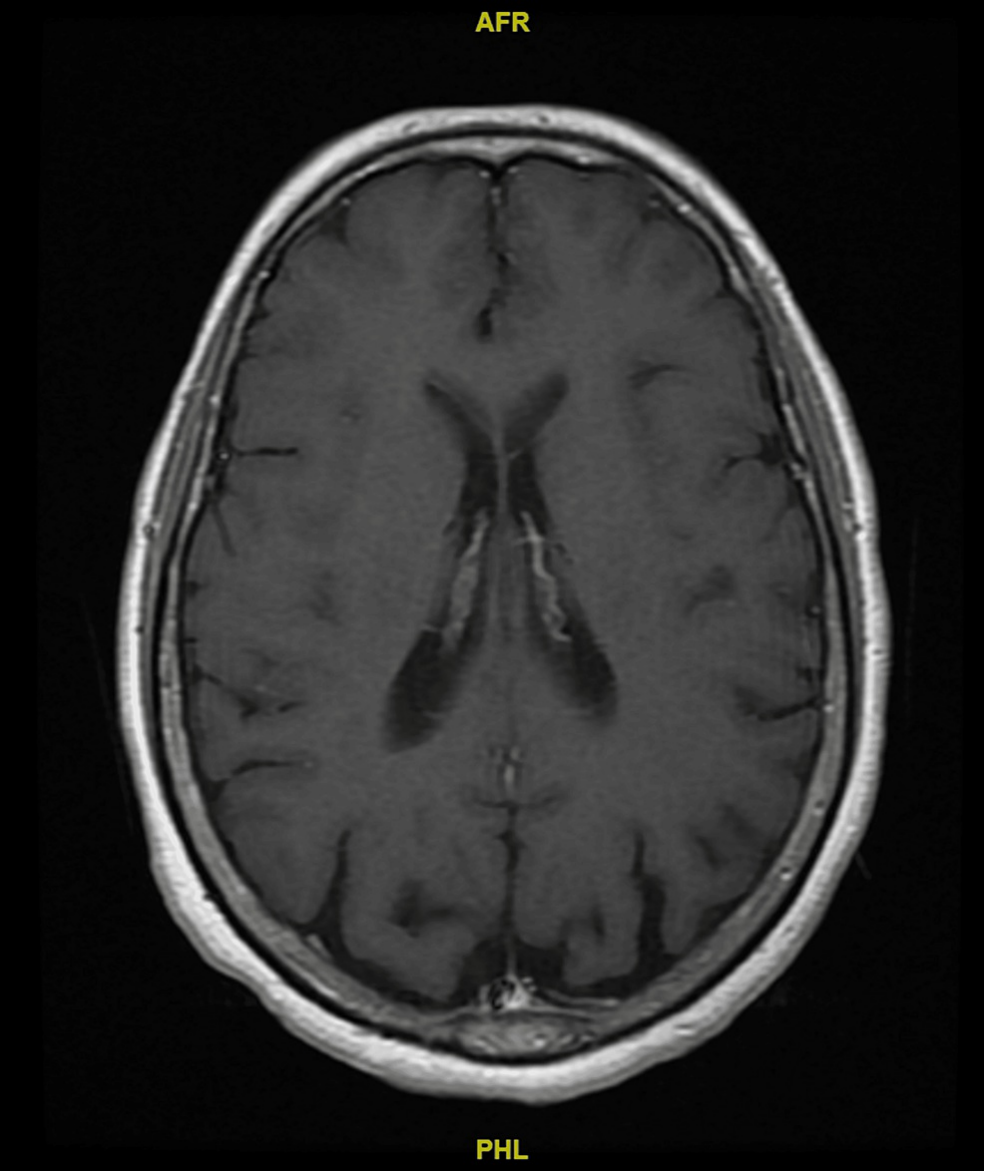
Non-contrast cerebral MRI showing dilatation of CSF spaces more than expected for the patient's age and nonspecific white matter changes in both cerebral hemispheres MRI: magnetic resonance imaging, CSF: cerebrospinal fluid
